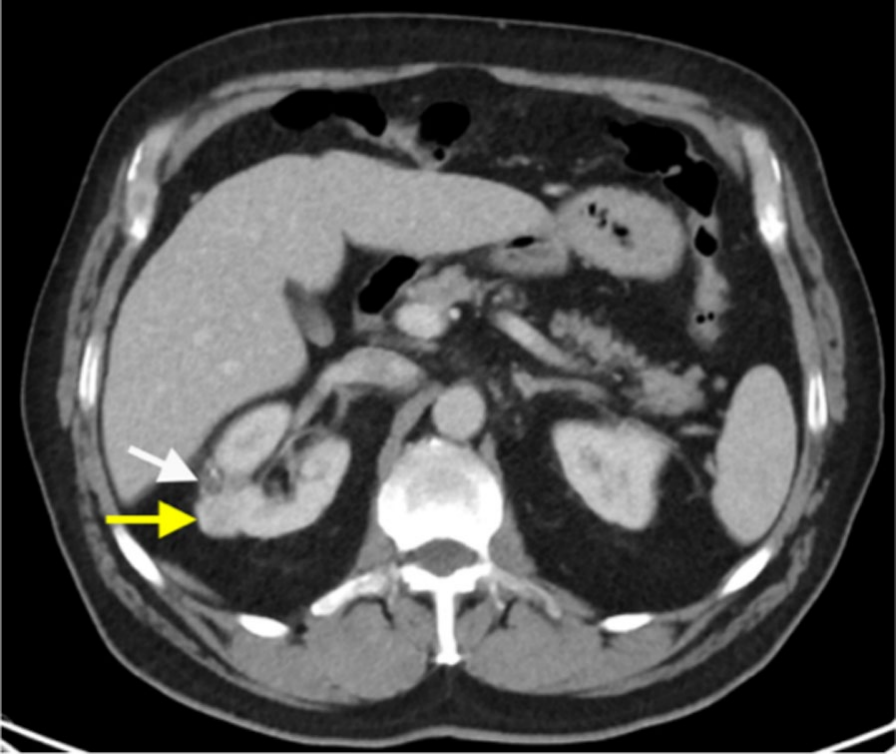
Axial contrast-enhanced CT image of recurrent renal cell carcinoma at a partial nephrectomy site at the mid-pole of the right kidney, as demonstrated by nodular enhancement (yellow arrow) at the site of the partial nephrectomy, usually hypodense (white arrow)
